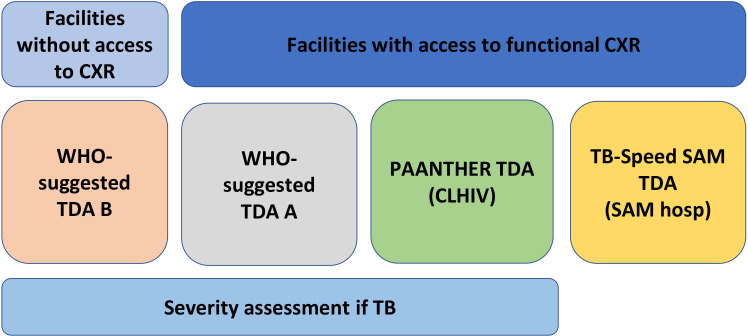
TDAs used according to entry sites and populations (PHC or DH). TB Speed SAM TDA will be used in children <5 years hospitalised with SAM. PAANTHER will be used in CLHIV<15 years. WHO TDA A or WHO TDA B will be used in children <15 years or children <10 years depending on the country adaptation. CLHIV, children living with HIV; CXR, chest X-ray; DH, district hospital; hosp, hospitalised; PHC, primary health clinic; SAM, severe acute malnutrition; TB, tuberculosis; TDA, treatment decision algorithm.
